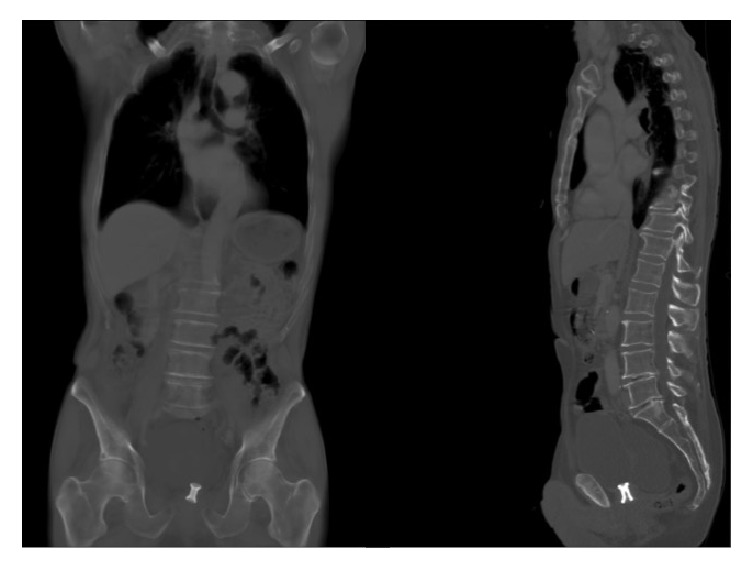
Frontal and lateral view of a CT (bone window) showing the double-cone Memokath 045 placed at the bladder neck.
